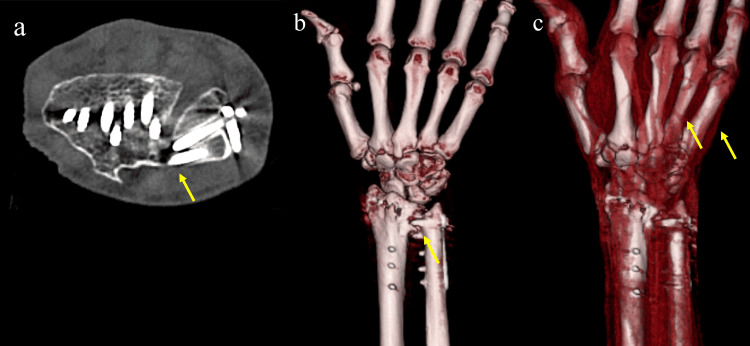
Computed tomography (CT) images: (a) axial CT image; (b) three-dimensional CT image; and (c) three-dimensional CT images of the extensor tendons.
